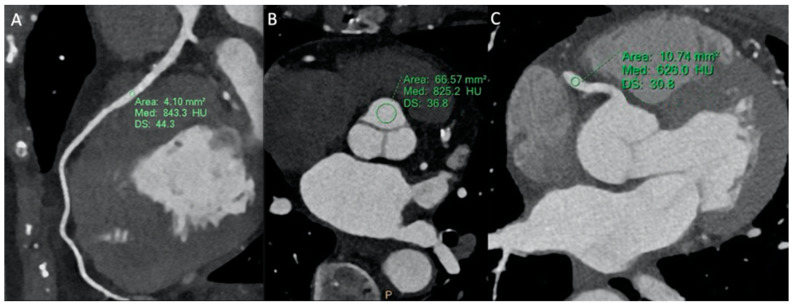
An 80 kVp 256-MDCT combined with the MBIR reconstruction technique. Low-dose coronary angiography study with the following dose values recorded: DLP: 27.1 mGy*m; CTDIvol: 2.1 mGy; and a calculated effective radiation dose (ED) of 0.7 mSv. Images of cardiac CT MBIR study with MPR reconstruction. (A) Curved reconstruction of left anterior descendant artery showing myocardial bridging, with good visualization of the artery wall due to high SNR and CNR. The ROI drawn in the intramyocardial tract shows a high attenuation value (843 HU) and low image noise (44 HU). (B) Aortic valve MPR reconstruction showing a good attenuation value (825 HU) and a low noise level (37 HU), allowing for an optimal evaluation of aortic sinuses. (C) Axial native image showing high attenuation value in the proximal tract of the right coronary artery.
